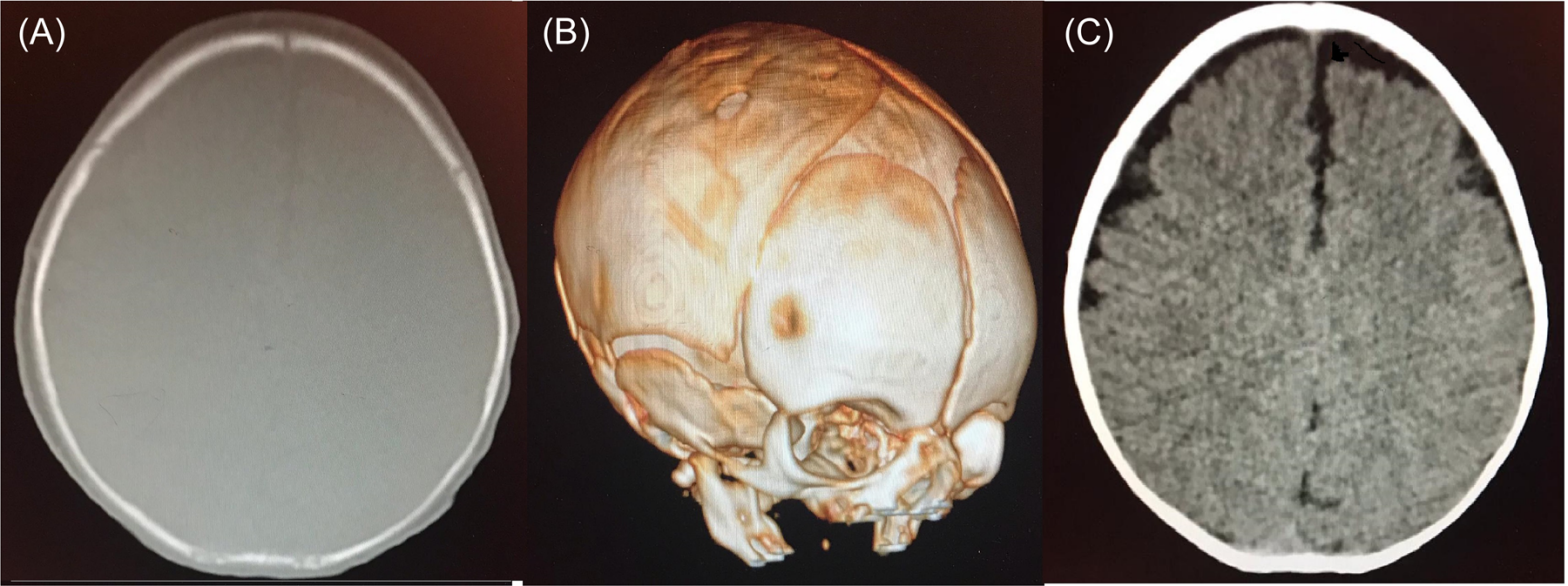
Postoperative (A) axial Computed tomography (CT) scan bone window, (B) 3D CT scan, and (C) plain CT scan images show remodeling of the calvarium with normal skull reconstruction and underlying brain.
